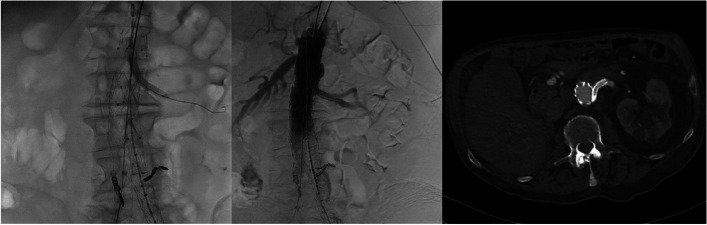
Chimney technique. Dilatation of the renal stent graft after deployment of the proximal aortic extension (DSA) (a). Elimination of type IA endoleak with a tubular extension and stent graft inserted into the solitary renal artery (DSA (b), CTA (c))
